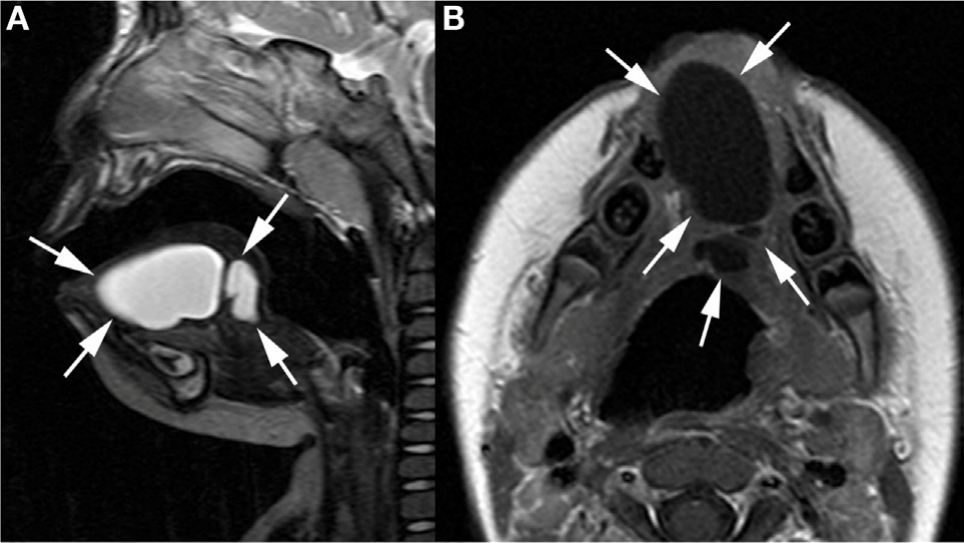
Sagittal T2-weighted (A) and axial gadolinium-enhanced T1-weighted image (B) show well-defined cystic lesion (arrows) in the midline of the oral tongue, septated in its posterior part.
